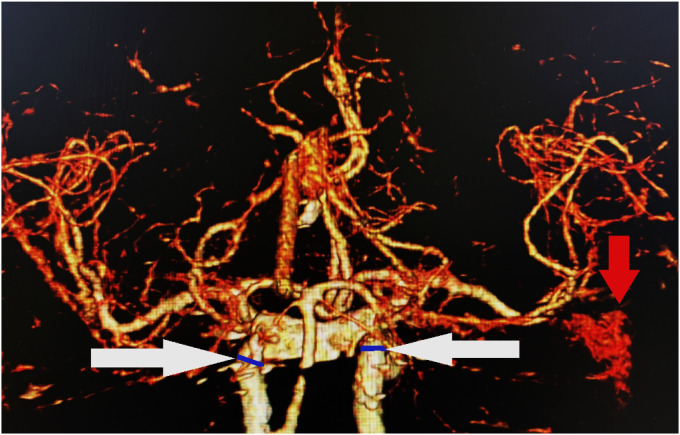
3D reconstruction of the multidetector computed tomography angiography (straight view). The white arrows point to the supraclinoid segment (C7) of both internal carotid arteries, where their radii were measured (marked with a blue line). The red arrow points to the parenchymal component of the intracerebral hemorrhage.
